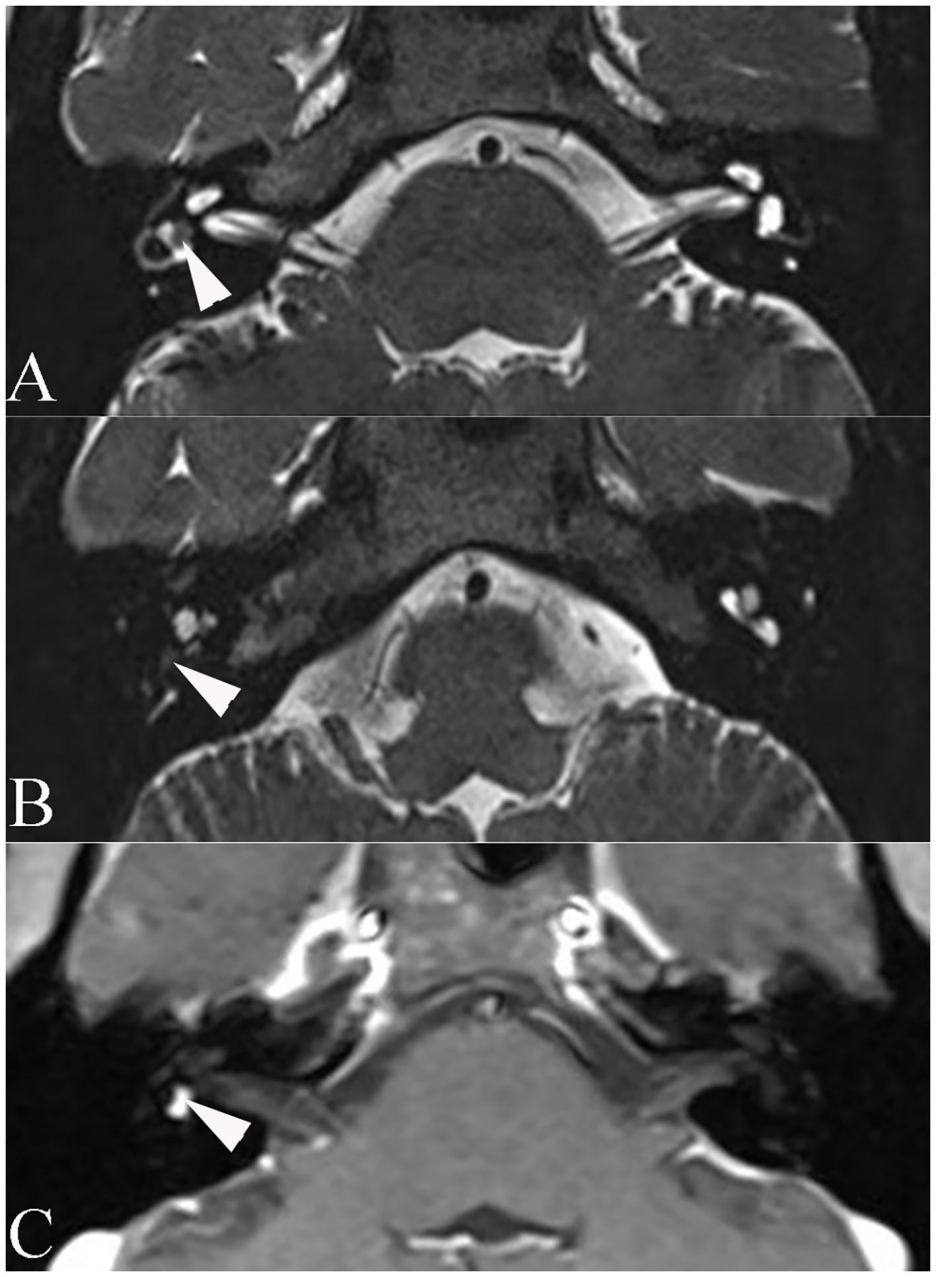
Axial magnetic resonance imaging of patient 6. (A) T2-weighted magnetic resonance imaging reveals a hypointense mass within the vestibule (arrowhead). (B) T2-weighted MRI reveals a hypointense mass within the basal turn of the cochlea (arrowhead). (C) Enhanced T1-weighted MRI reveals signal enhancement of the mass (arrowhead).
